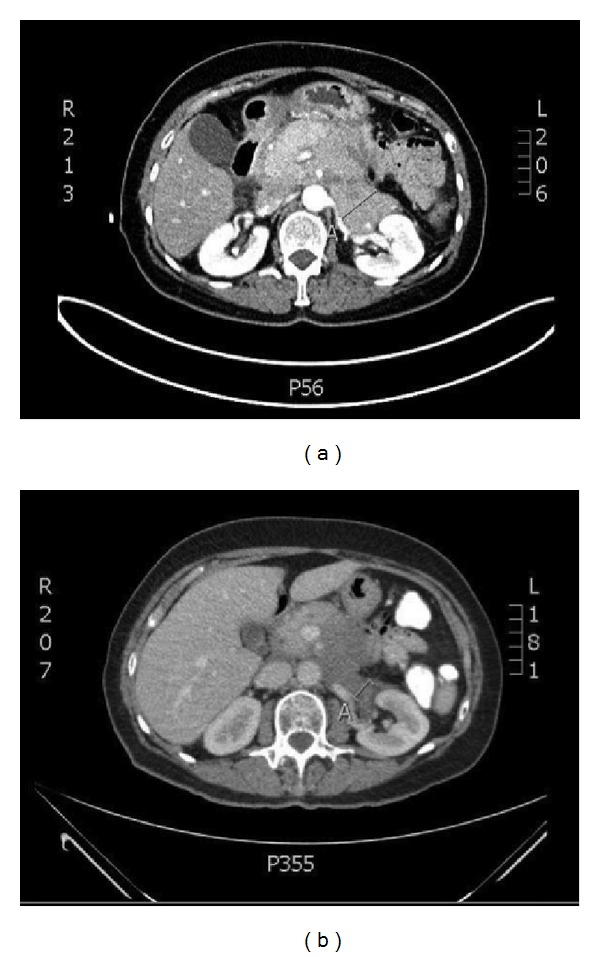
CT abdomen shows a confluent lymphoma mass commencing at the celiac trunk and extending inferiorly to the pelvic outlet (a) before and (b) after radiotherapy.
